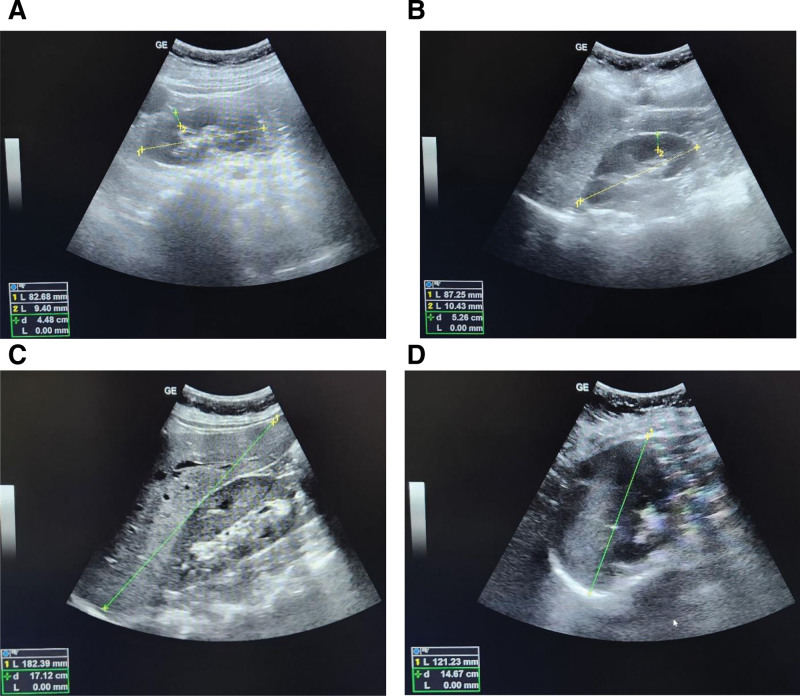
Ultrasonography images of the patient as described in Table 1. (A) Ultrasonography images of left kidney (8.27 cm showing normal echotexture). (B) Ultrasonography images of right kidney (8.72 cm showing normal echotexture). (C) Ultrasonography images of liver showing hepatomegaly (18.2 cm). (D) Ultrasonography images of spleen showing splenomegaly (12.1 cm).
